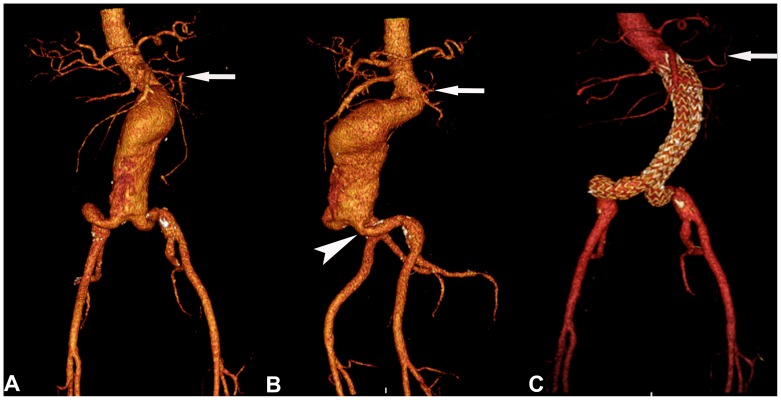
CTA of an infrarenal AAA in a 74-year-old patient. A, VR of the long aneurysm with a short proximal neck. The aneurysm involves the aortic bifurcation, and there is marked angulation of the infrarenal portion (arrow indicates the left renal artery). Both common iliac arteries are markedly elongated. B, Lateral VR more clearly showing the elongation of the left common iliac artery and also severe kinking (arrowhead) at its origin as well as marked infrarenal angulation of the proximal neck (arrow indicates the left renal artery). C, Postinterventional VR indicating successful implantation of an Endurant stent graft and exclusion of AAA (arrow indicates the left renal artery).
